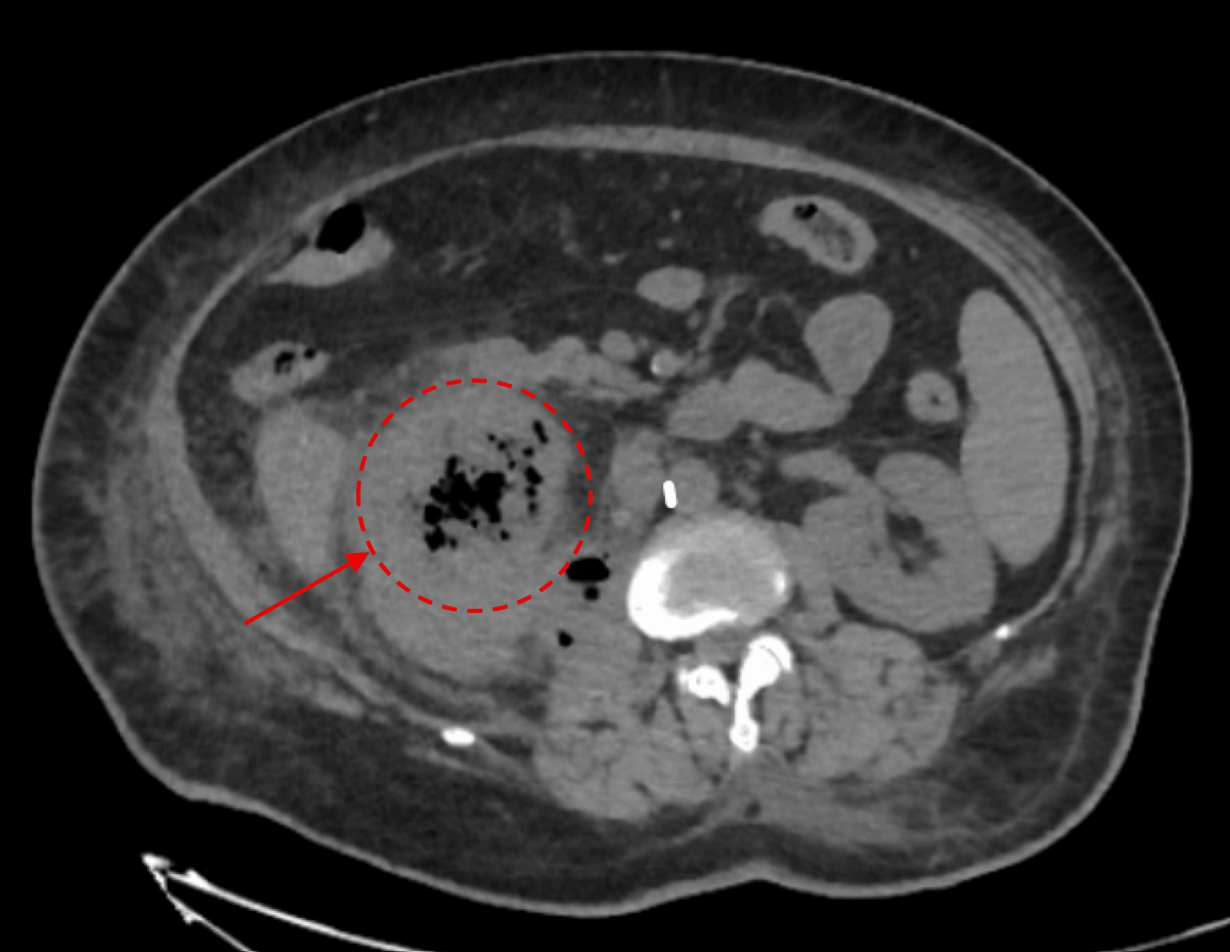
CT scan demonstrating the multiloculated nature of the retroperitoneal abscess (marked with a red arrow), consistent with the need for percutaneous drainage
